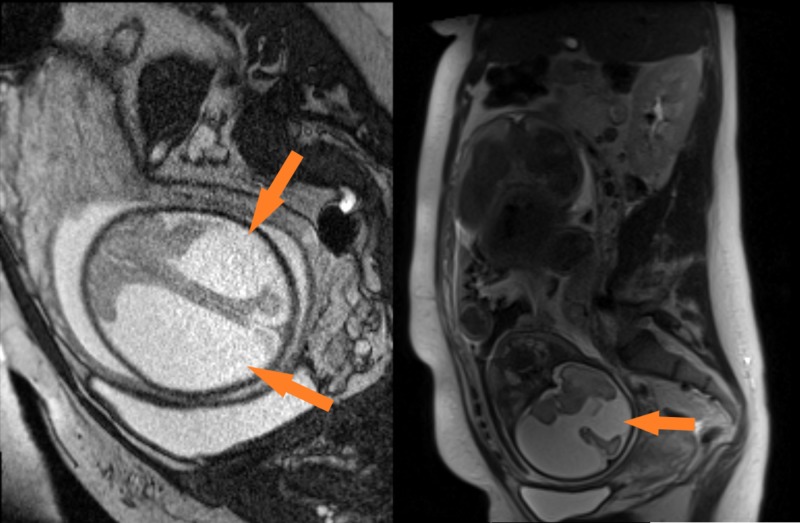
Fetal MRI at 28 weeks Images from fetal MRI at 28 weeks showing porencephaly in bilateral middle cerebral artery territories.
